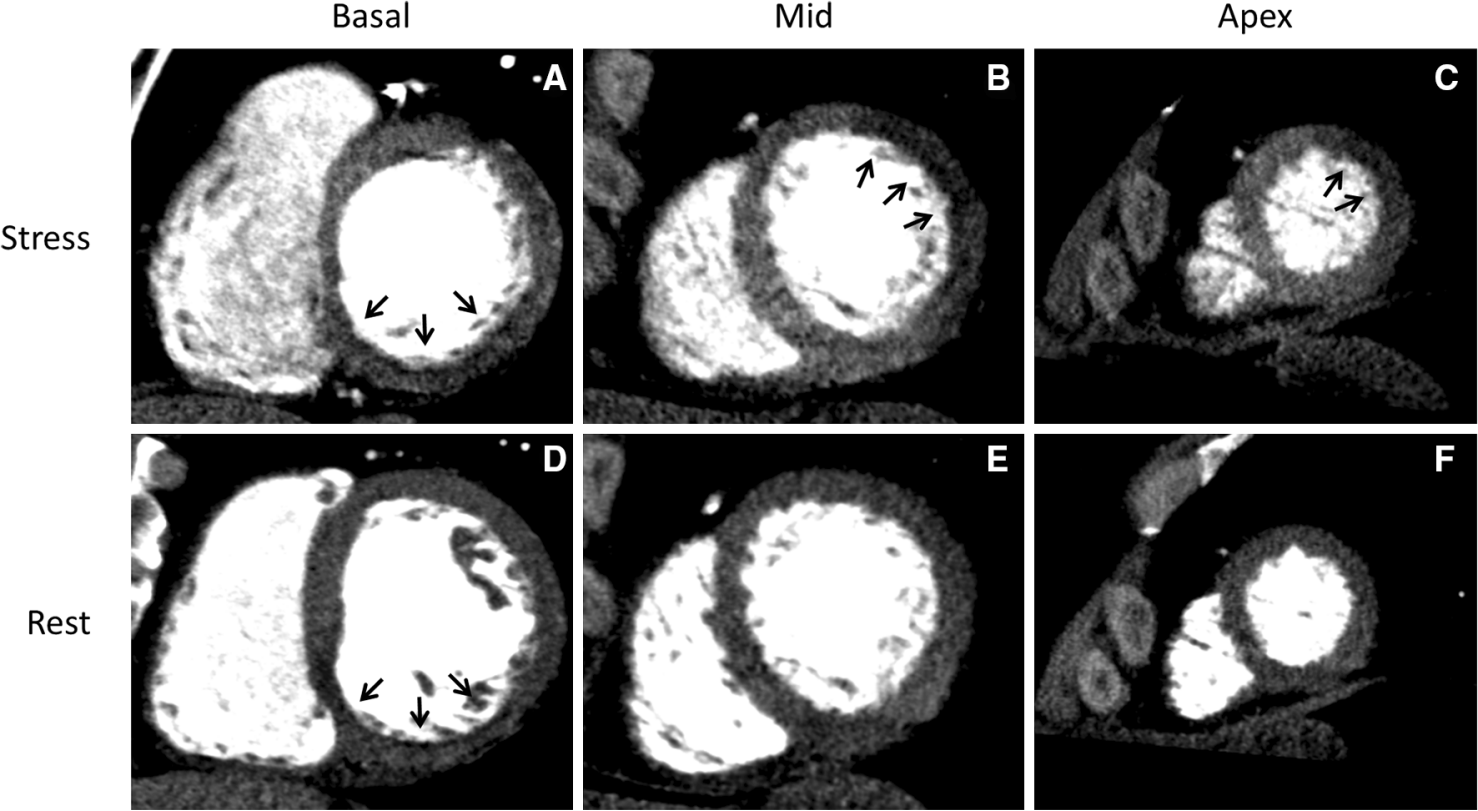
Short-axis views of CTP images at stress and rest. (A, D) At the basal level, hypo-enhancement in the inferior wall (*arrows*) is depicted at rest and stress indicating prior myocardial infarction. (B, C) At stress, hypo-enhancement in the anterolateral wall (*arrows*) at mid and apical level. (E, F) Normal myocardial enhancement at rest. *CTP*, myocardial computed tomography perfusion
